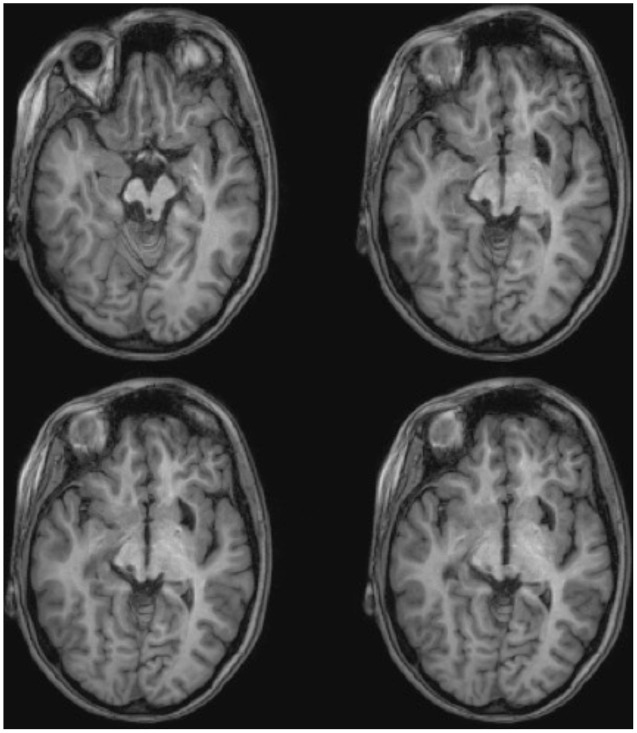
High resolution (0.7 mm^3^) T1-weighted MRI of patient ML. Axial slices from ventral (Top left) to dorsal (Bottom right) showing damage to the left inferior colliculus and mesencephalic tegmentum ventral and lateral to the SC.
